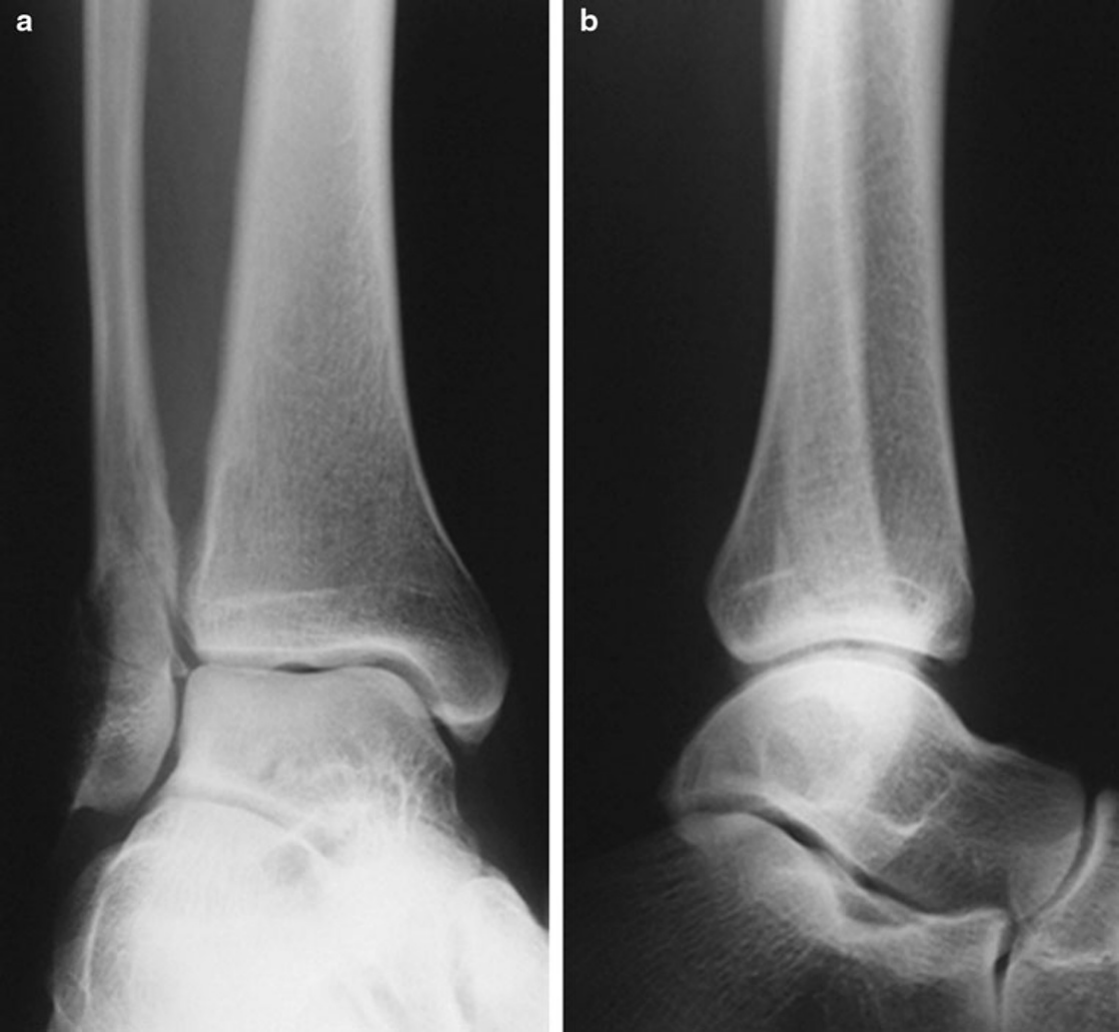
AP and lateral radiographic images of a SE-2 fracture, consisting of a spiral or oblique fibula fracture at the level of the syndesmosis
